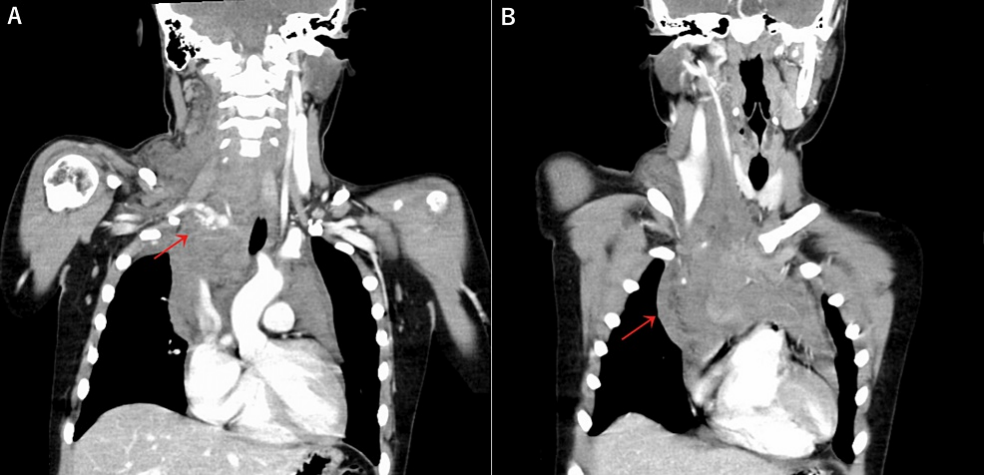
Chest contrast-enhanced CT on arrival (A) Active bleeding from the proximal portion of the right subclavian artery. (B) Hematoma in the mediastinum.
